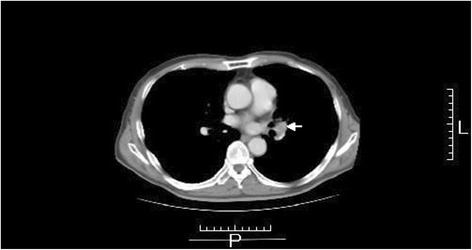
Chest computed tomography on 31 August 2010. Swelling of a lymph node, 20 mm in size, is recognized at the hilum of the left lung (*arrow*)
